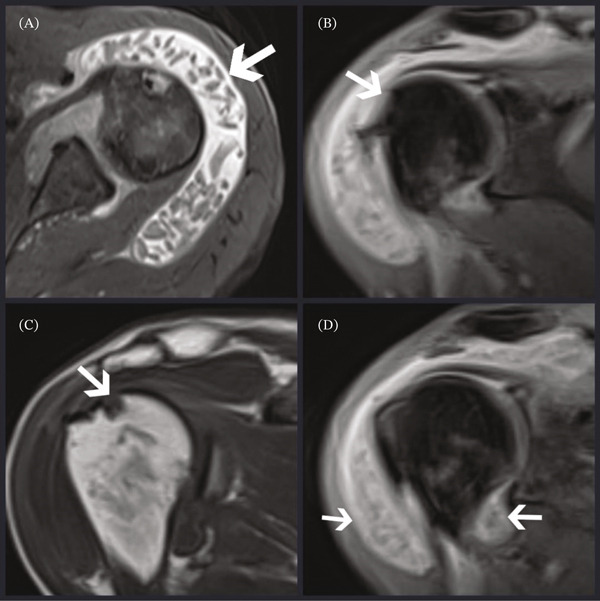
MRI findings. (A) Coronal T2‐weighted fat‐suppressed image showing multiple hypointense rice bodies within the subacromial–subdeltoid bursa (arrow). (B) Axial T2‐weighted fat‐suppressed image demonstrating a full‐thickness supraspinatus tendon tear (arrow). (C) Coronal T1‐weighted image revealing focal bone erosion at the humeral head (arrow). (D) Axial T2‐weighted fat‐suppressed image depicting diffuse synovial thickening and joint effusion (arrows).
